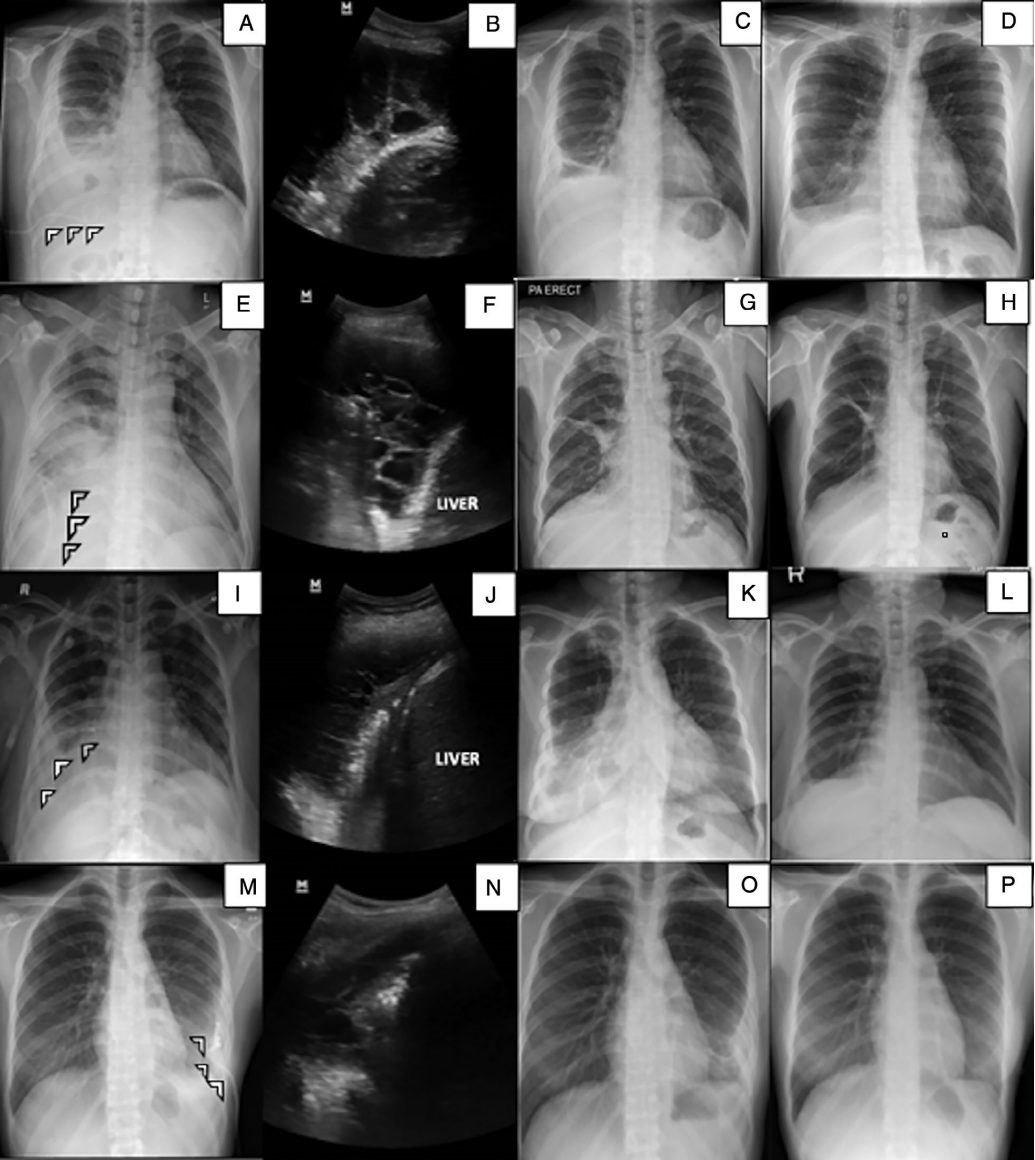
Chest radiographs on initial presentation, showing unilateral pleural effusion in case 4 (A), case 5 (E), case 6 (I), and case 7 (M); Intercostal chest catheter in situ (arrows). Ultrasound thorax revealed multiloculated effusion in case 4 (B), case 5 (F), case 6 (J), and case 7 (N). Chest radiographs day 7 post intrapleural alteplase–deoxyribonuclease (DNase) and two months post treatment showed residual effusion and residual pleural thickening, respectively, in case 4 (C, D), case 5 (G, H), case 6 (K, L), and case 7 (O, P).
